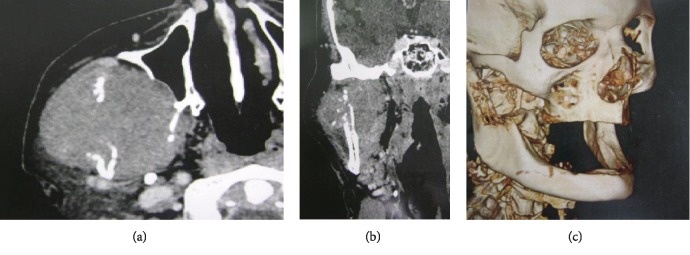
(a) Axial CT image reveals an expansive lesion in the soft tissue of mixed aspect and regular contours. (b) Coronal CT image shows hyperdense foci among the lesions. (c) 3D reconstruction of CT scan reveals mandibular ramus lytic lesions.
